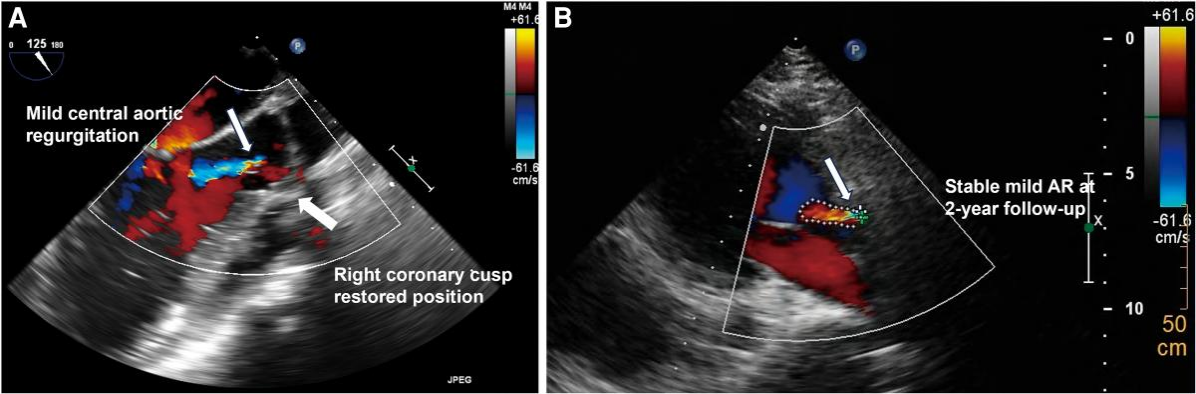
Post-repair and follow-up echocardiographic assessment. (*A*) Intraoperative transoesophageal echocardiography immediately after valve-sparing repair demonstrating restored cusp coaptation with mild residual central aortic regurgitation. (*B*) Transthoracic echocardiography at 2-year follow-up showing stable mild aortic regurgitation without progression.
